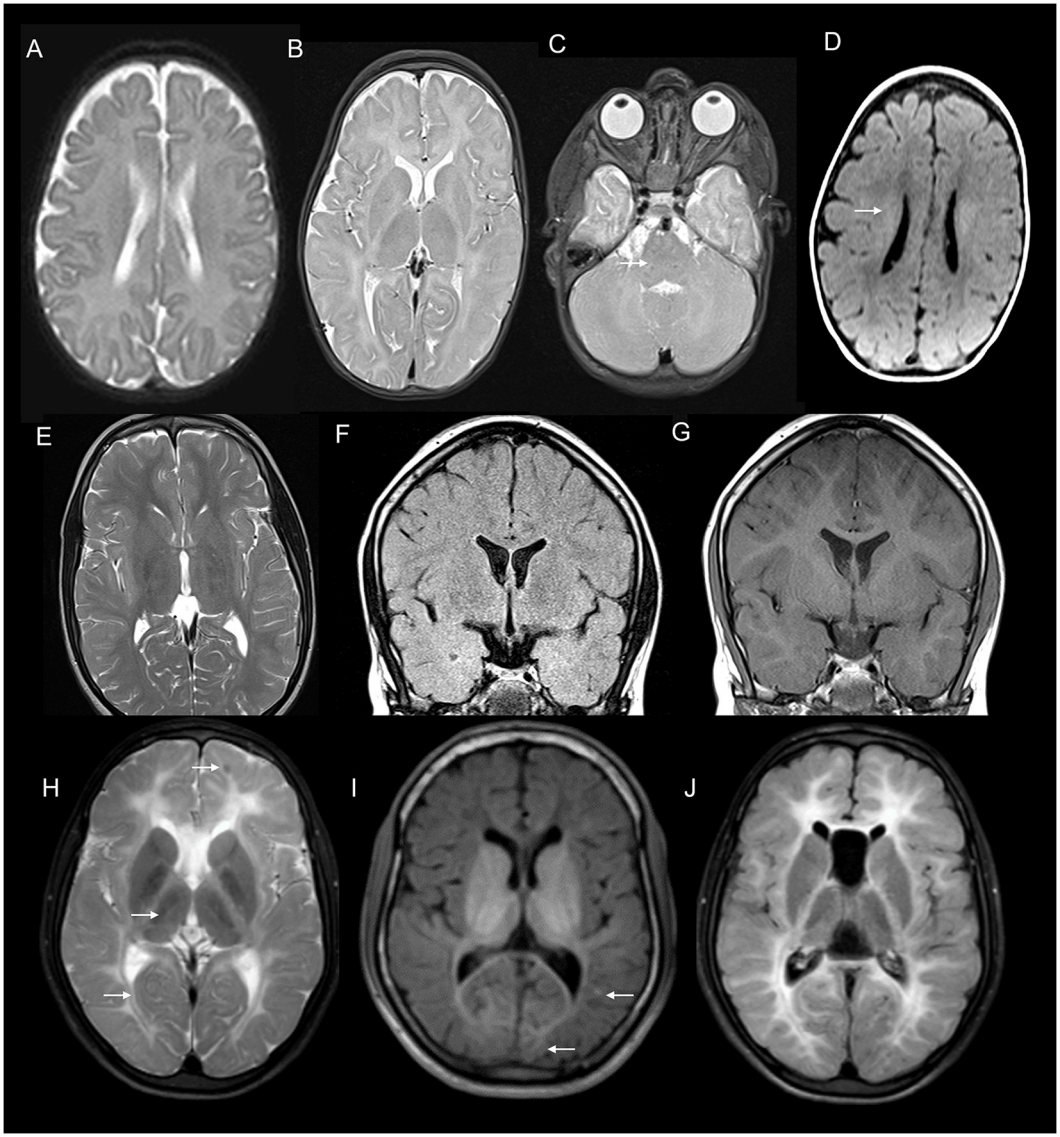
MRI features of hypomyelinating leukodystrophies. MRI features of an adolescent with spastic ataxic syndrome due to PMLD with T2 axial at 1 year (A) and 7 years (B, C) show near complete absence of myelination on the initial scan with no progression on the second scan. Note the diffuse pontine involvement in image C which is more common in PMLD than PMD.T1 axial image (D) shows hypo intensity of the white matter except in centrum semiovale. 18 q-syndrome in a 15 year old; T2 axial (E) shows reduced myelination than expected for age with a washed out appearance on FLAIR (F) but the preservation of hyperintensity on T1 images (G). Combination of these signal abnormalities on various sequences is highly suggestive of hypomyelination. MRI brain findings of an individual with *POLR3*-related hypomyelination with T2 (H), T1 (I), and FLAIR (J) axial images showing diffuse hypomyelination with sparing optic radiations and patchy myelin islands. T2 also shows hypo intensity of ventrolateral thalamus due to the relative preservation of myelin.
